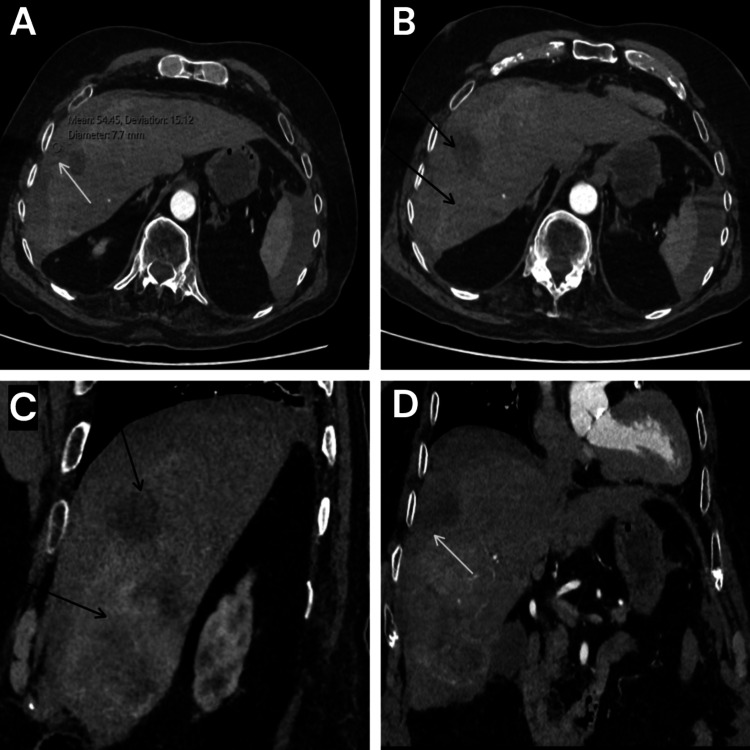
Contrast-enhanced CT of the aorta showing subcapsular hepatic hematoma and hepatic metastases (A) Axial CT view demonstrating high-density fluid in the subcapsular space of the right hepatic lobe, consistent with capsular breach and subcapsular hematoma (arrow). (B) Axial CT view showing multiple peripherally enhancing hepatic lesions consistent with metastases (arrows). (C) Sagittal CT view demonstrating multiple peripherally enhancing hepatic lesions consistent with metastases (arrows). (D) Coronal CT view demonstrating capsular breach with associated subcapsular hematoma (arrow).
